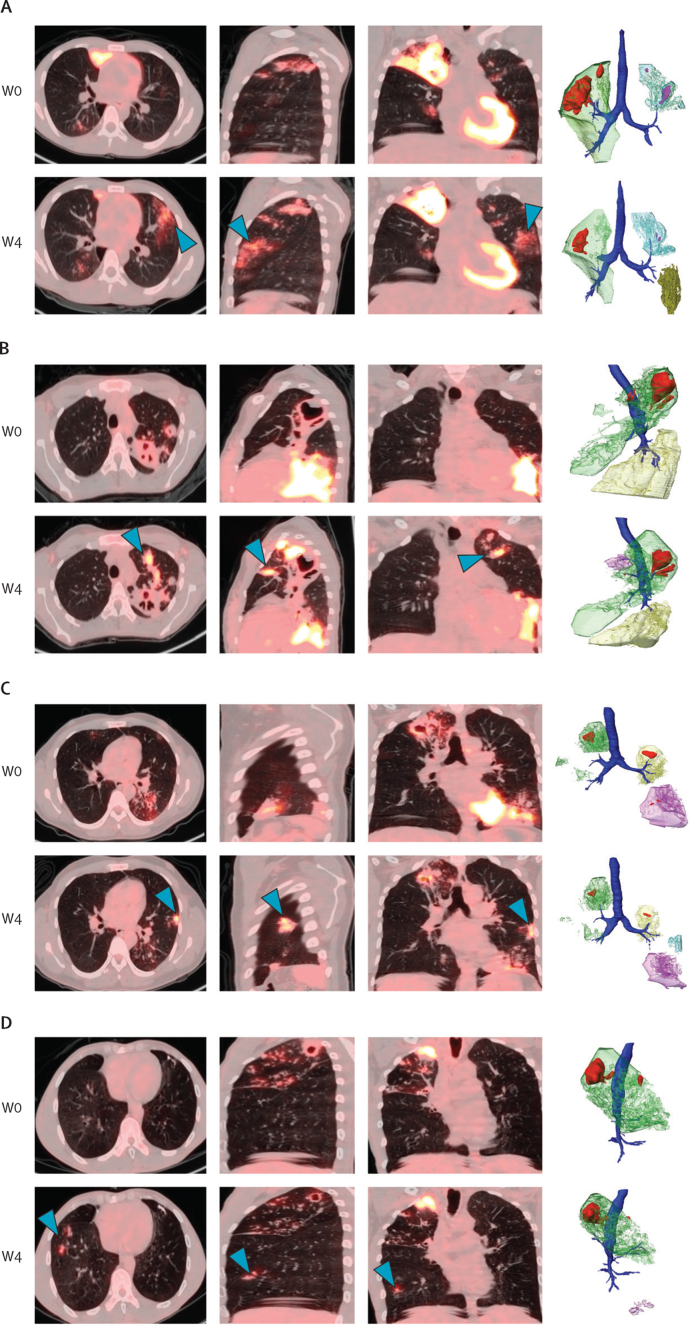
Representative examples of new lesions on the W4 PET-CT scan with 3D renderings (A) Large right upper lobe cavity (red) with surrounding dense consolidation (green) and smaller left segments 1–3 cavities (pink) with surrounding consolidation (blue). All cavities shrank by W4. There was a new left side lingular infiltrate (blue arrows, green-brown lesion on 3D rendering) at W4 that was not present at W0. (B) At baseline, there was a large posterior left upper lobe S1–2 cavity (red) with small anterior S3 cavity (red) at baseline and associated consolidation (green) primarily in left S1–2 down to the lingula S4–5. There was also an extensive left lower lobe consolidation (yellow) as well. At W4, left S1–2 segment cavity had decreased in size but with markedly increased PET uptake around it. Left lower lobe consolidation, however, had improved. There was a new left S3 segment consolidation (blue arrows, pink lesion on 3D rendering) that was not present at baseline. (C) At baseline, there were cavities (red) with associated consolidations in the right upper lobe, apex of the left lower lobe, and in the left base. At W4, all of these had improved but there was a new left lingular lesion (blue arrows, blue lesion on 3D rendering) that was not present at baseline. (D) At baseline, disease was confined to the right upper lobe with a S1 cavity, surrounding consolidation, and spread of disease to the S3 segment. At W4, there were two new foci of nodular disease (blue arrows, purple lesion on the 3D rendering) in the right lower lobe S8 segment. 3D=three-dimensional. W=week.
